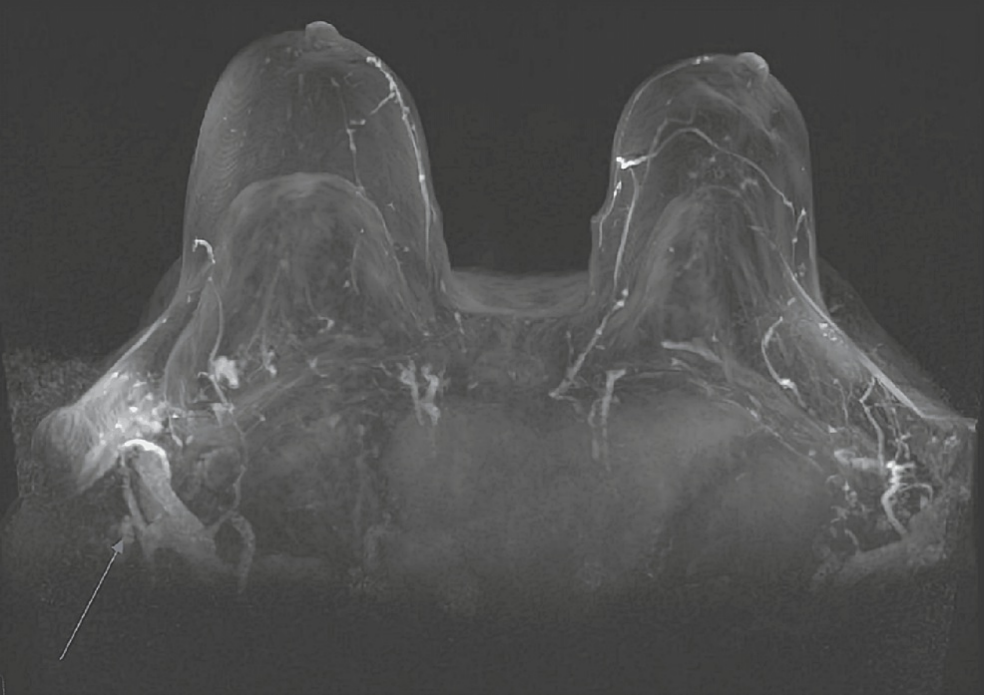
MRI of the breast was remarkable for right breast implant rupture with silicone lymphadenitis (White arrow)
